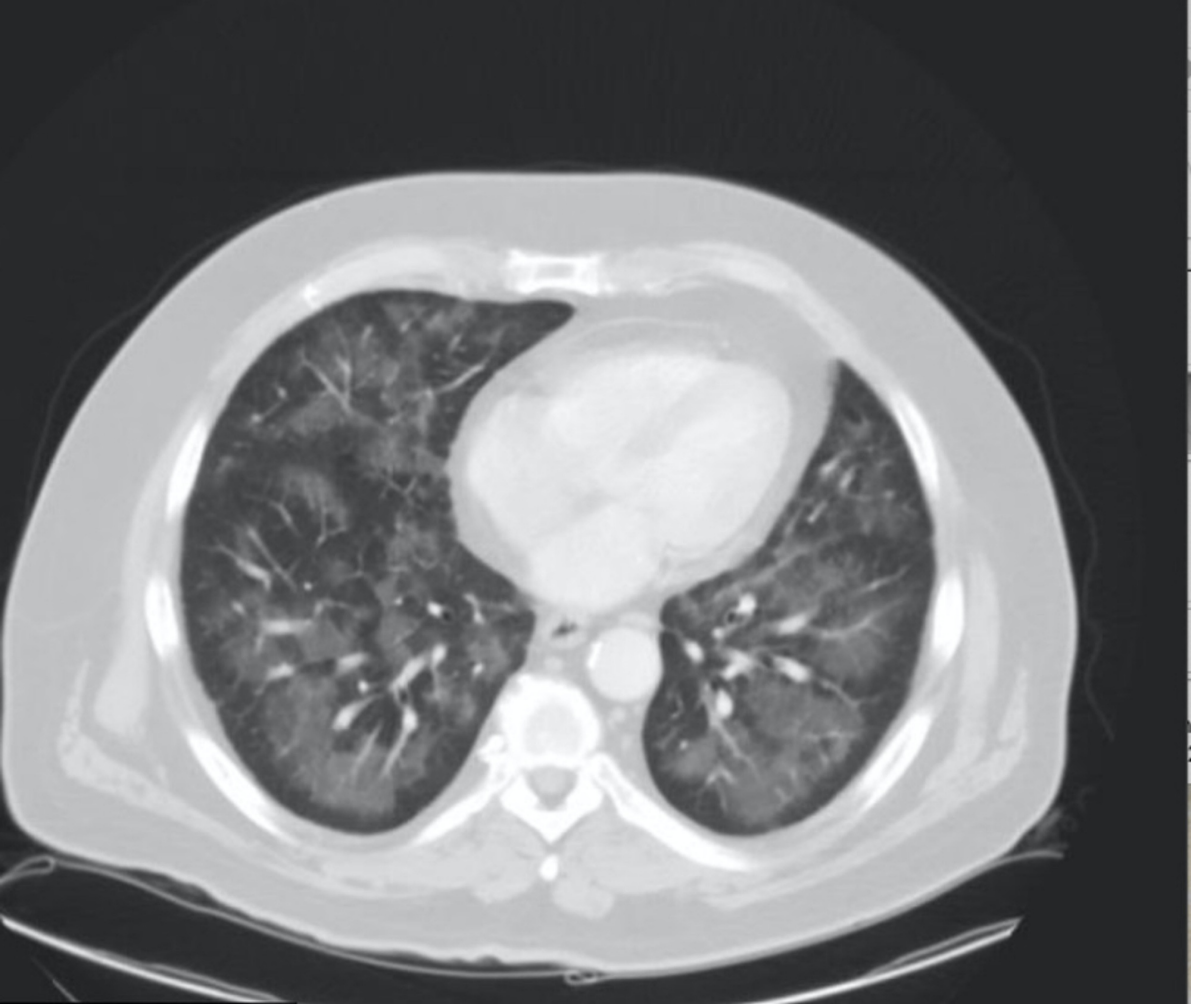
CT chest on the first emergency visit showing bilateral ground-glass opacities, likely pneumonia or edema CT: computed tomography
